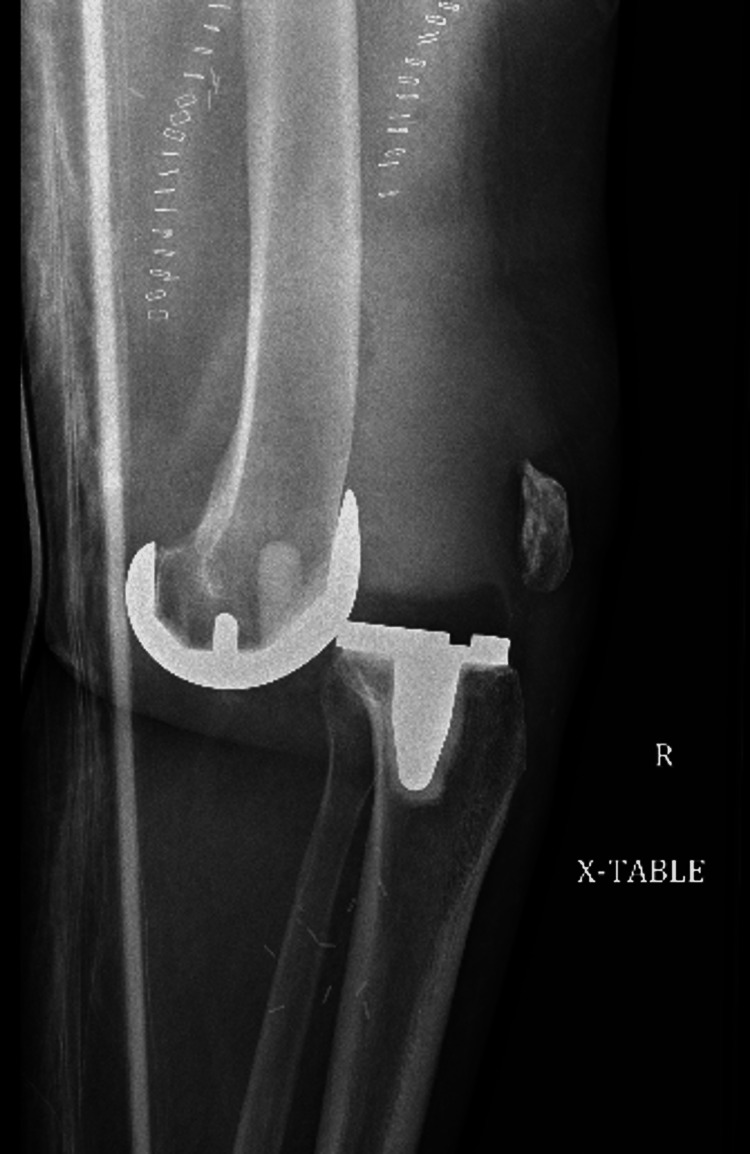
Postoperative day 0 X-ray showing anterior re-dislocation of the joint.
